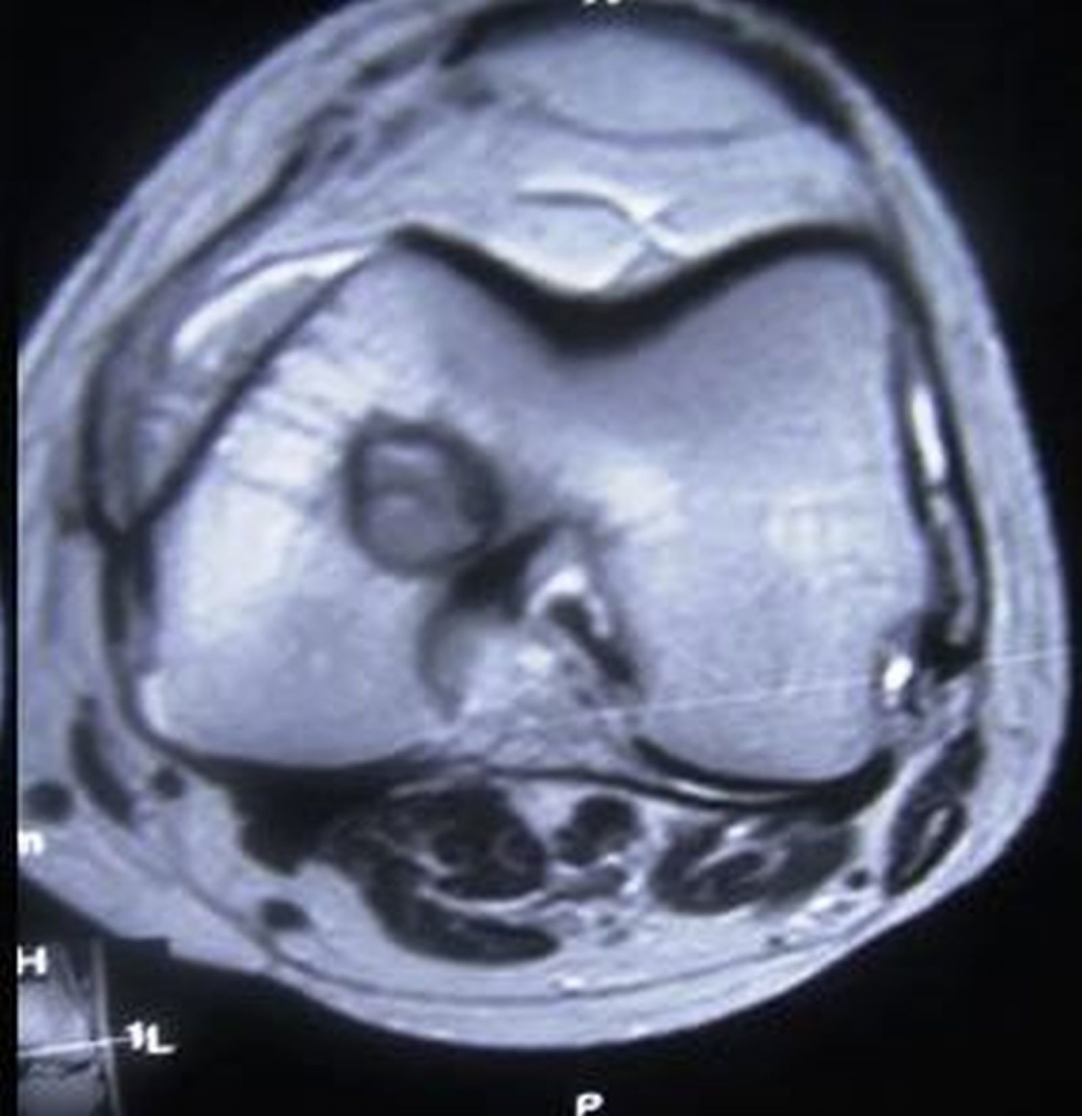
MRI axial section showing increased signal intensity of the lesion
